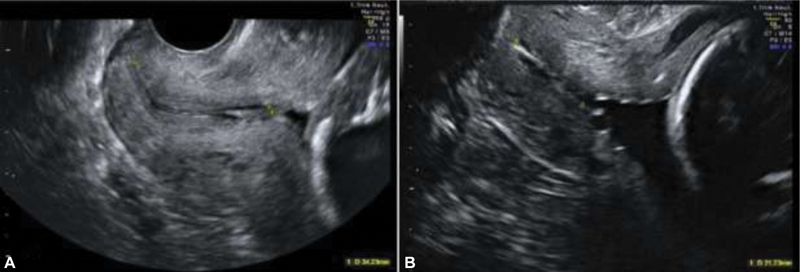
Transvaginal ultrasound measurement of the cervical length (TUCL). (
